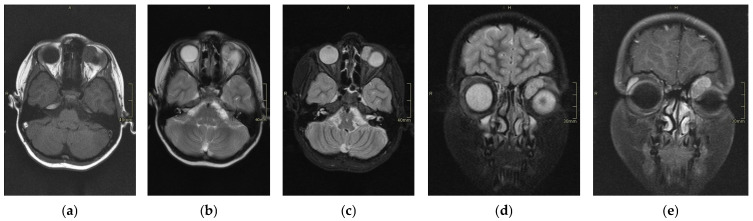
Magnetic resonance imaging (MRI) of RMS case: T1 axial section (a), T2 (normal) axial section (b), T2 with fat saturation axial section (c), T2 fat saturation coronal section (d), and T1 post-gadolinium (e). This case represents hypothesis T1, hyperintense T2, and intense enhancement postcontrast (gadolinium).
